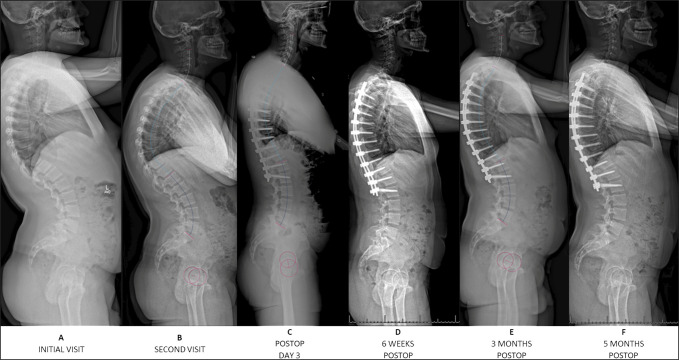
Standing lateral radiographs from the initial visit to 5 months postoperatively. A and B, Preoperatively, the patient had severe thoracic kyphosis. C, Radiographs show posterior-only multilevel Smith-Peterson osteotomies from T7 to T11 and pedicle screw instrumentation and fusion from T4 to L2. D–F: Postoperative radiographs obtained at 6 weeks, 3 months, and 5 months.
